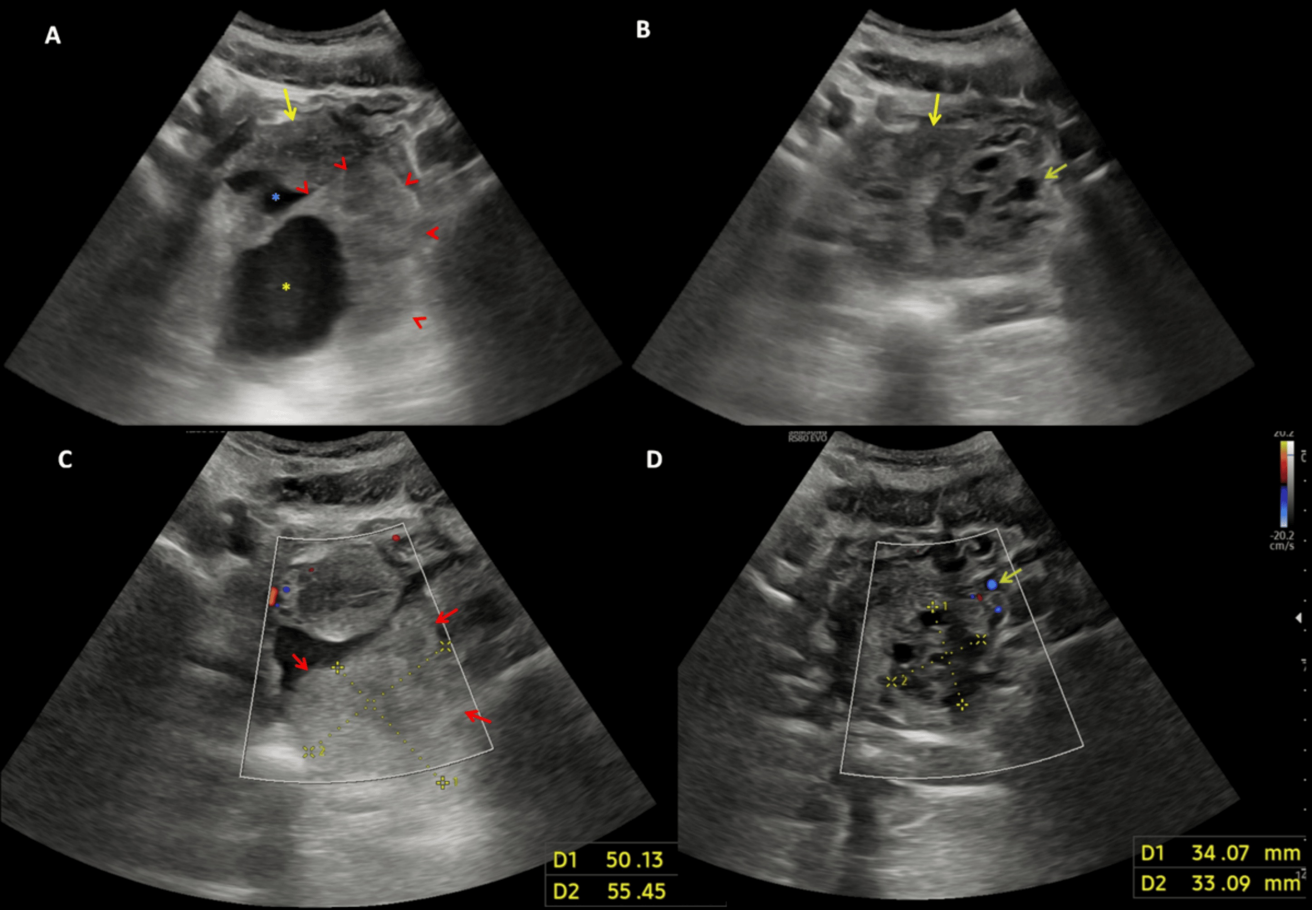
Ultrasound assessment of the pelvic mass A. Ultrasound image demonstrating a midline retro-uterine pelvic mass (red arrowheads), slightly deviated to the left, with a reniform shape and heterogeneous echotexture. The mass contains an internal cystic component (yellow asterisk) and is associated with a moderate volume of free pelvic fluid (blue asterisk). The uterus exhibits normal morphology (yellow arrow). B. Corresponding image delineating the uterus (yellow arrow) and a normal-sized left ovary with a follicular pattern (green arrow). C & D. Color Doppler imaging reveals an absence of vascular flow within the pelvic mass (red arrows), whereas the left ovarian vascular pedicle demonstrates preserved perfusion (green arrow). The right ovary and its vascular pedicle are not visualized.
